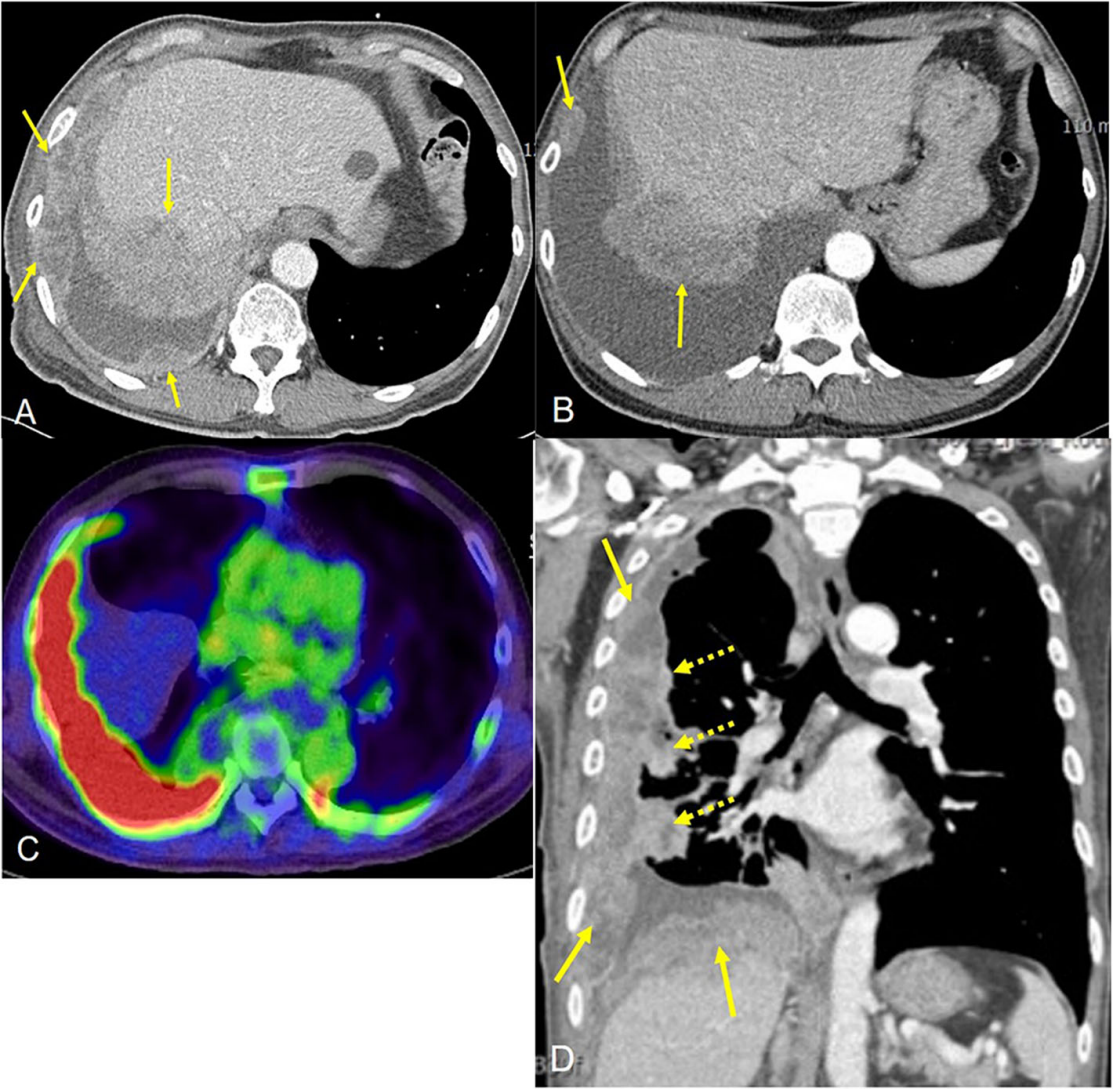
Enhanced multidetector CT (A, B) of a 67-year-old man showed right circumscribed, necrotic pleural masses. PET-CT(C) showed very intense uptake at the right pleural masses. 2D enhanced coronal reformat image (D) showed right visceral (dashed arrows) and parietal pleural masses(arrows)
